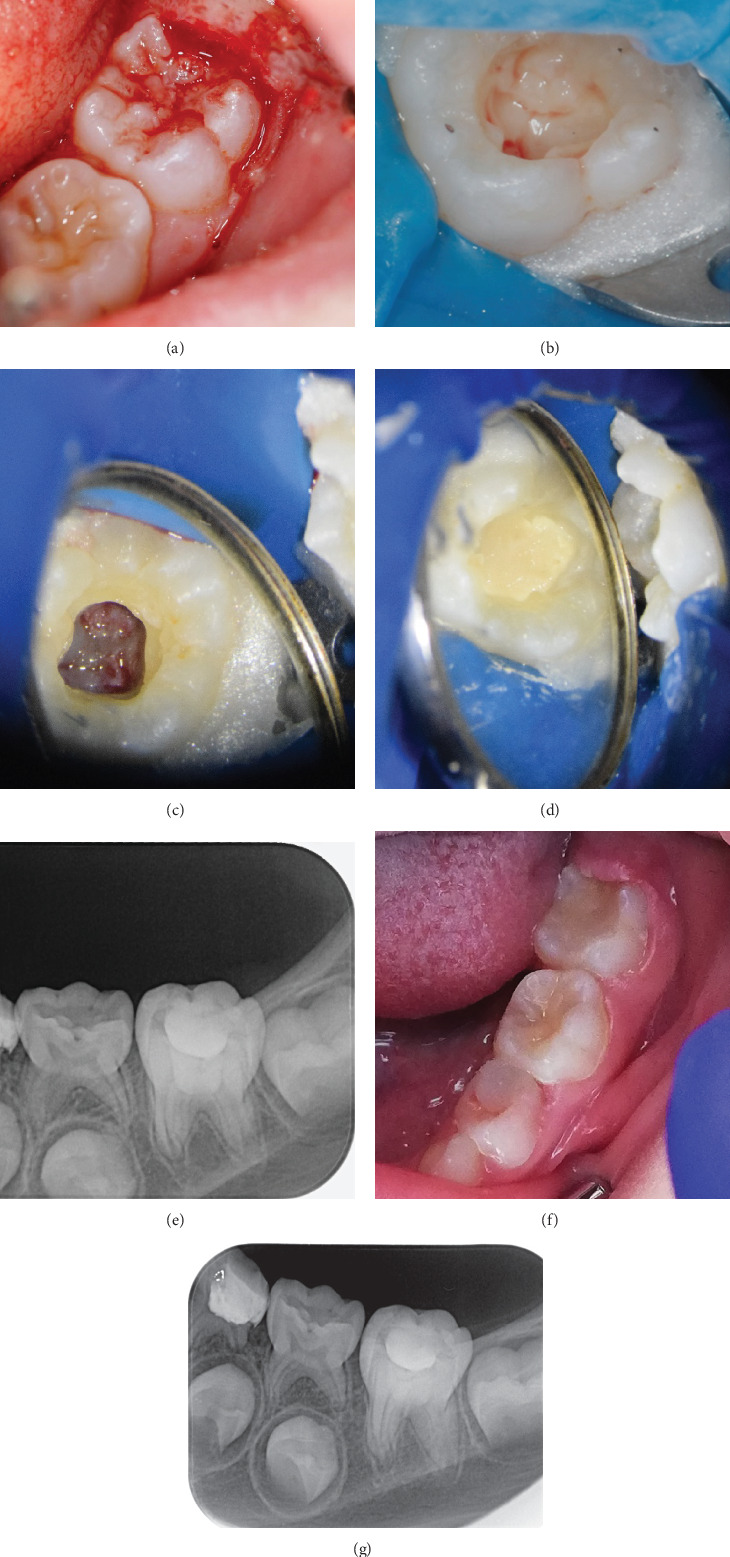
(a) Intraoral image after gingivectomy; (b) pulp exposure in the mesiobuccal pulp horn; (c) image after coronal pulpotomy and hemostasis; (d) applied Biodentine and glass ionomer cement; (e) postoperative control radiograph; (f) condition of the soft tissue after 1 week; and (g) 6 months follow-up.
